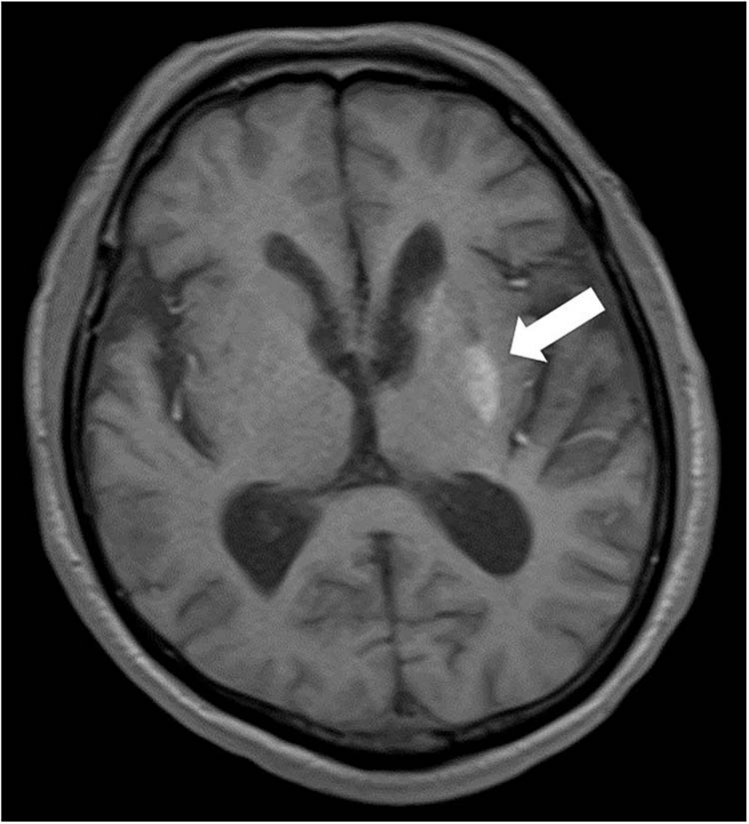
T1 MRI showed hyperintensity in the left basal ganglia (arrow) during patient’s second admission.
